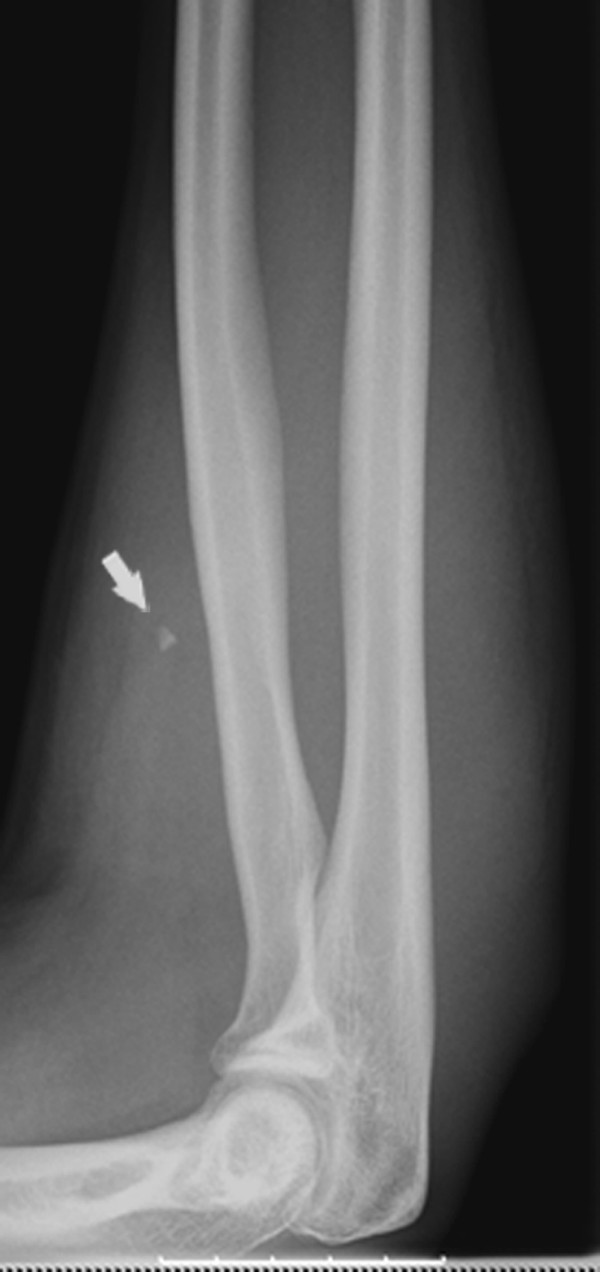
X-ray. The arrow shows the glass-like foreign body in the forearm.
